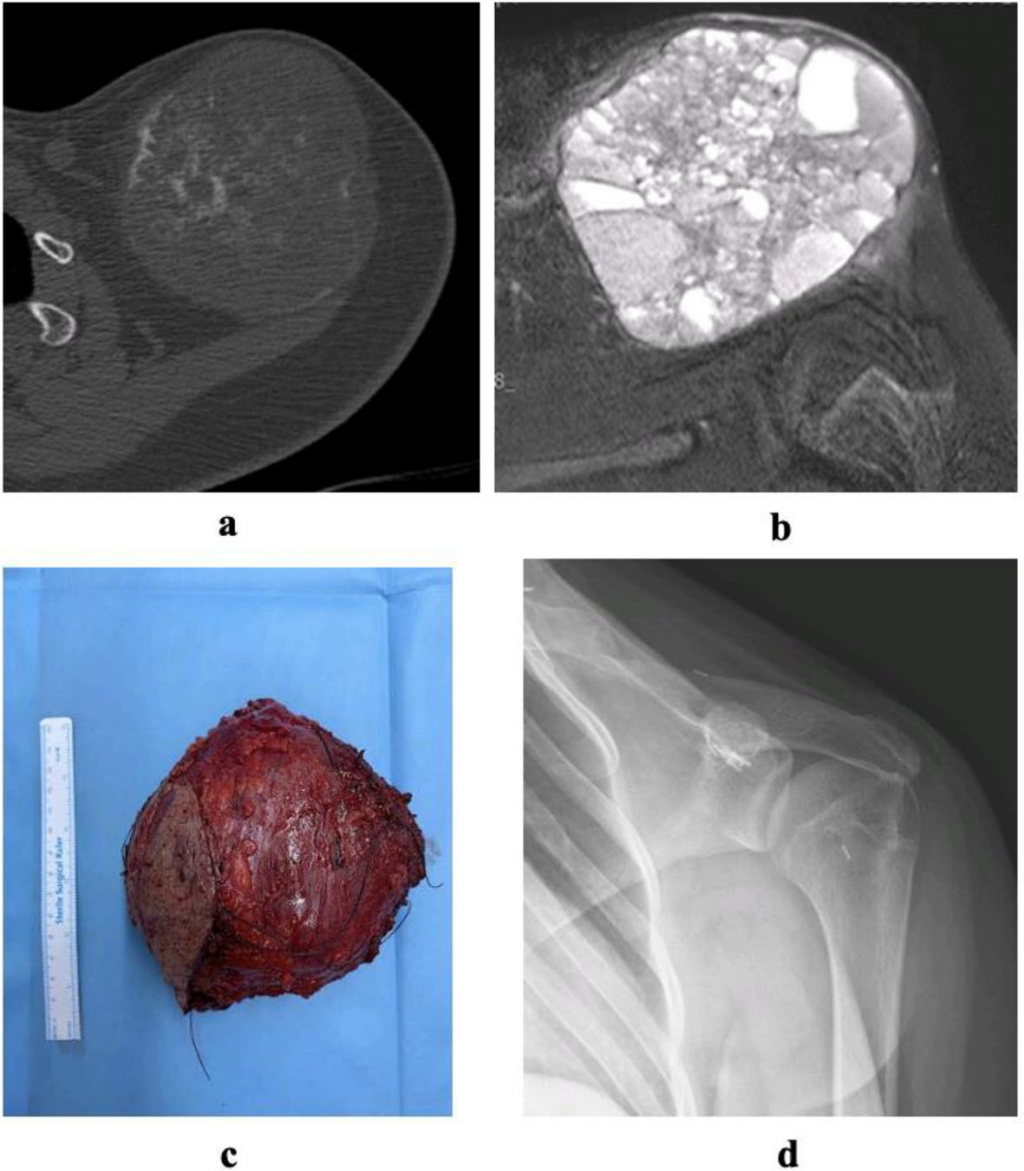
a CT axial section of aneurysmal bone cyst located in the clavicle. b MRI axial section of the cyst. c Peroperative resection material. d Postoperative radiograph after resection
